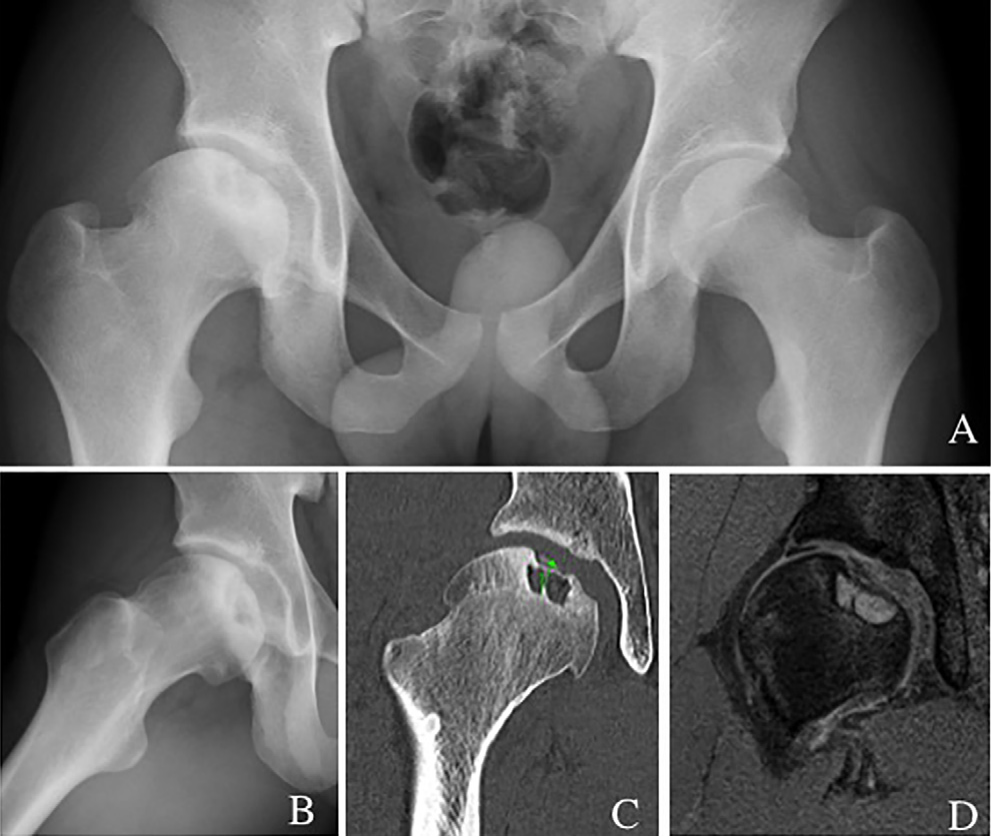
(A and B) Preoperative anteroposterior radiograph and modified Dunn view (45° flexion and 20° external rotation) showing OCD lesion of the aspherical shaped femoral head. (C) CT showing OCD lesion including subchondral cystic lesion of the femoral head. (D) MRI showing OCD lesion and subchondral cystic change.
